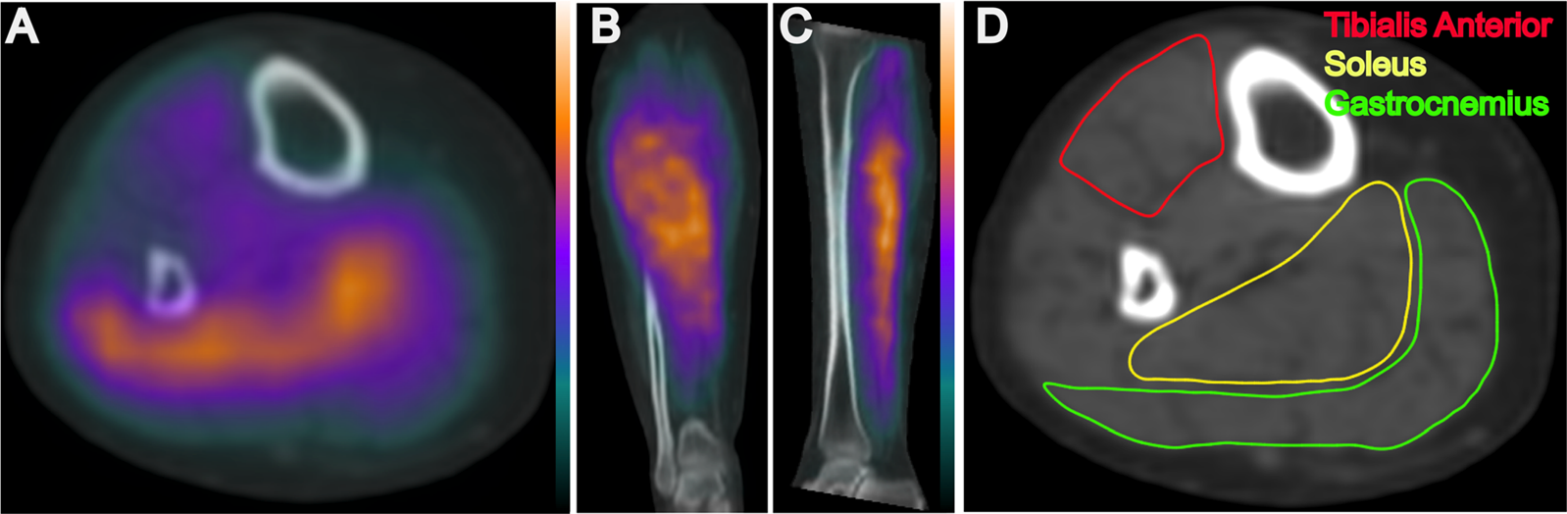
SPECT/CT imaging of lower extremity perfusion and CT-based image segmentation for regional perfusion analysis. Representative A transaxial, B coronal, and C sagittal views of SPECT/CT perfusion imaging of the calf. D Image segmentation of specific calf muscles of interest from a low-dose CT attenuation scan
